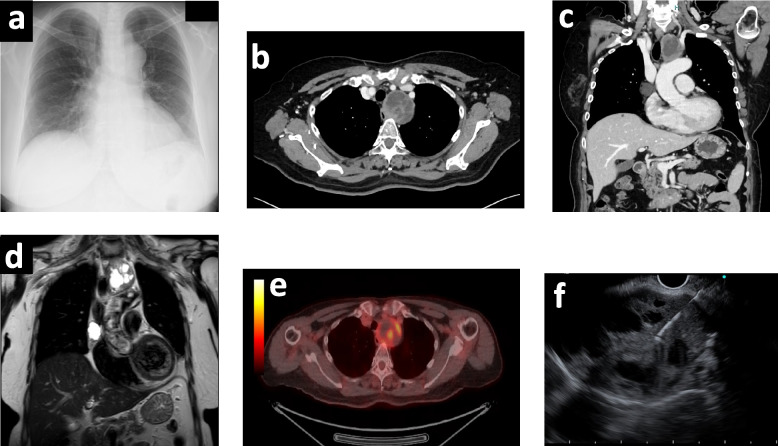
Preoperative imaging. a Upright chest radiograph showing mediastinal widening without diaphragmatic paralysis. b–c Contrast-enhanced CT: solid, well-circumscribed mass in the left tracheoesophageal groove and a right anterior mediastinal cystic lesion. d Contrast-enhanced MRI: encapsulated solid tumor with multiloculated cystic components and a separate mediastinal cyst. e 18F-FDG PET: uptake in the solid lesion (SUV_max 4.89). f Transesophageal EUS-guided FNA: spindle cells compatible with schwannoma
